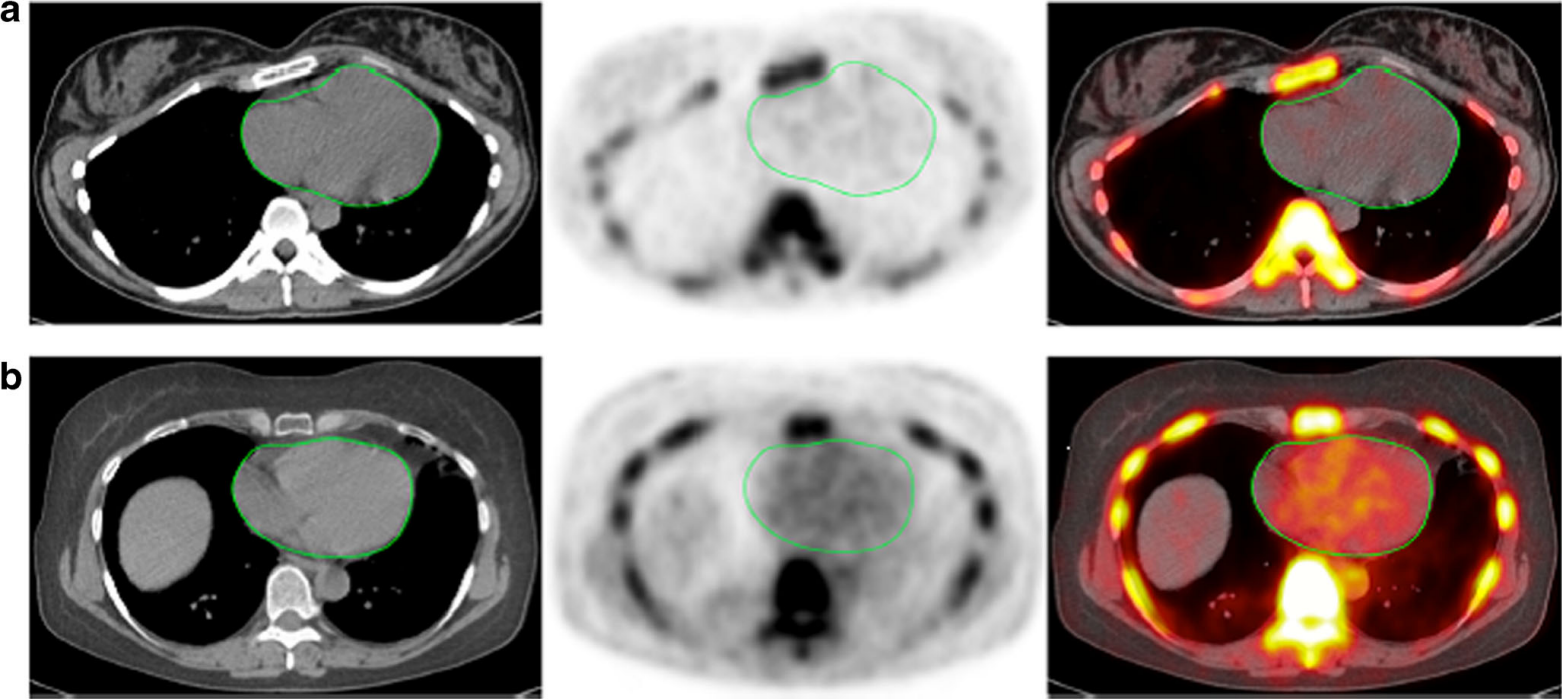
Transverse images (*left* CT, *middle* PET, *right* PET/CT) of the heart (*green circles*) in two clinically normal subjects (a 25 years old, b 61 years old). The global cardiac calcification scores were 12,492.44 in subject a and 18,424.70 in subject b. Normalizing the values to background NaF uptake increases the discrepancy between the subjects, resulting in 2.18 times the uptake in subject b than in subject a. Corresponding to the sites of NaF uptake in subject b, no structural calcification is seen on the corresponding CT scan and there is significant disparity between the PET and CT results. This is not an uncommon observation in this setting and clearly demonstrates the basis for assessment of cardiovascular calcification with these two different imaging modalities. While molecular imaging with NaF detects the earliest evidence for vascular calcification, evidence for calcification on CT largely reflects an end-stage disease process and therefore may be an irreversible pathologic state. Disparity between these two observations provides evidence for stage of calcification and has implications for the irreversibility of macrocalcification
